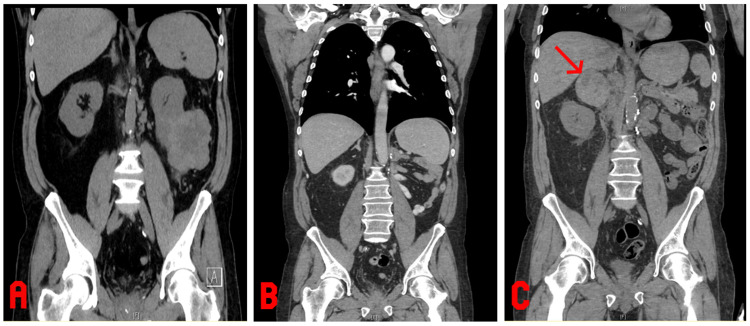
Computed tomography images. (A) Computed tomography of the abdomen/pelvis: noncontrast, coronal view, left-sided RCC; (B) Computed tomography of the chest/abdomen/pelvis: noncontrast, coronal view, post-left adrenalectomy and nephrectomy; (C) Computed tomography of the abdomen/pelvis: noncontrast, coronal view, new tumor invasion in the right adrenal gland with hemorrhage (red arrow). RCC: renal cell carcinoma
